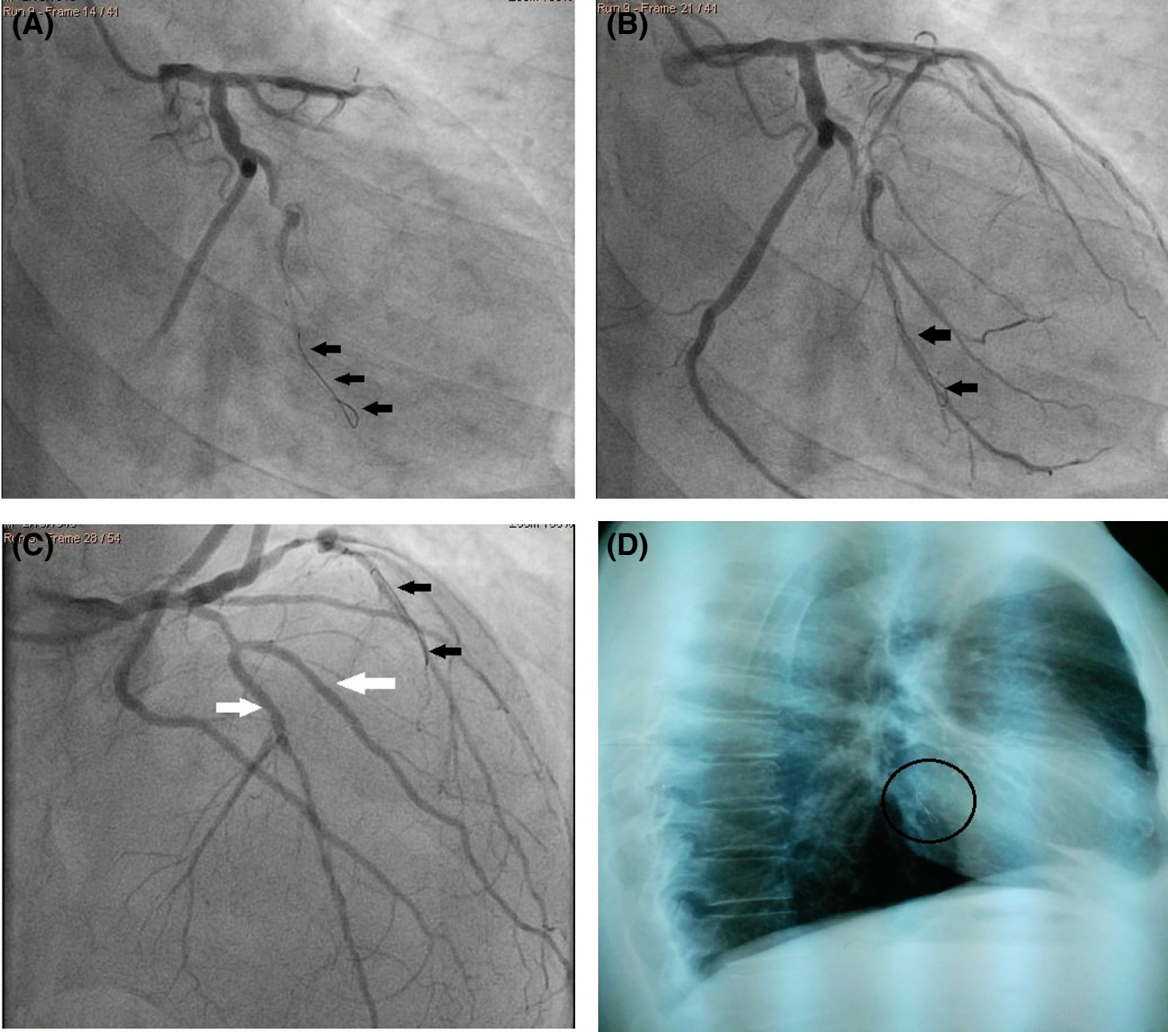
Coronary angiography and Chest radiography: (A and B) Coronary angiography: fractured guide wire in the first obtuse marginal branch (OM_1_) (black arrow), (C) Coronary angiography: fractured guide wire in the first obtuse marginal branch (OM_1_) (black arrow) and with white arrow noted the left anterior descending artery (LAD) and the first diagonal branch (D_1_). (D) Chest radiography (profile view) demonstrates a fractured guide wire in the black circle.
